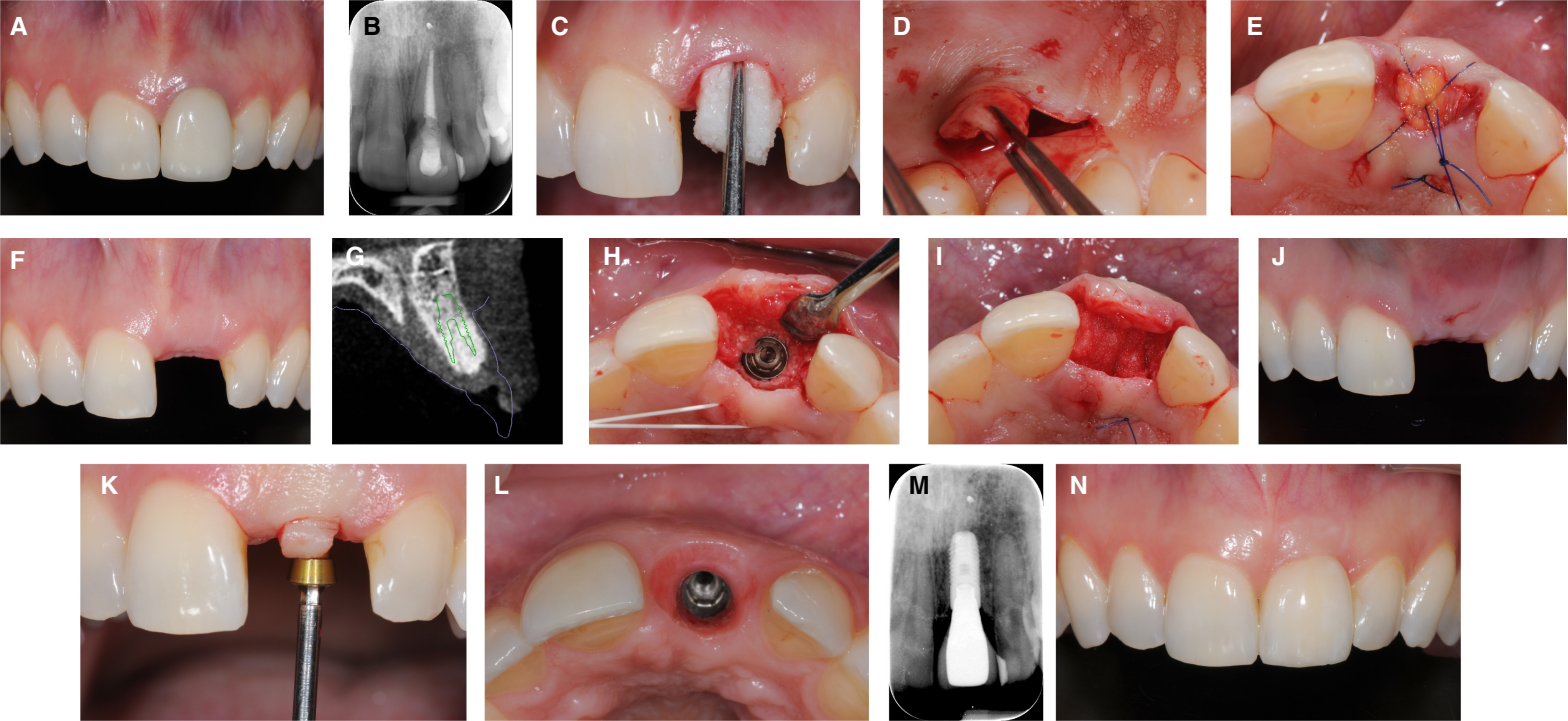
Clinical case of soft tissue management simultaneous to ridge preservation and in combination with implant placement. A, Patient's initial situation. B, Periapical radiograph of tooth 21. C, Alveolar ridge preservation. D, Palatal sub‐epithelial connective tissue graft. E, Sealing of the socket in ridge preservation. F, Post‐operative healing after 6 months. G, Cone‐beam computed tomography scan of healed site 21. H, Implant placement. I, Soft tissue grafting with volume‐stable xenogeneic collagen matrix. J, Post‐operative healing after 2 weeks. K, Abutment connection with U‐Flap. L, Emergence profile of implant 21. M, Periapical radiograph of osseointegrated implant 21. N, Patient's final situation after receiving the implant supported‐restoration
